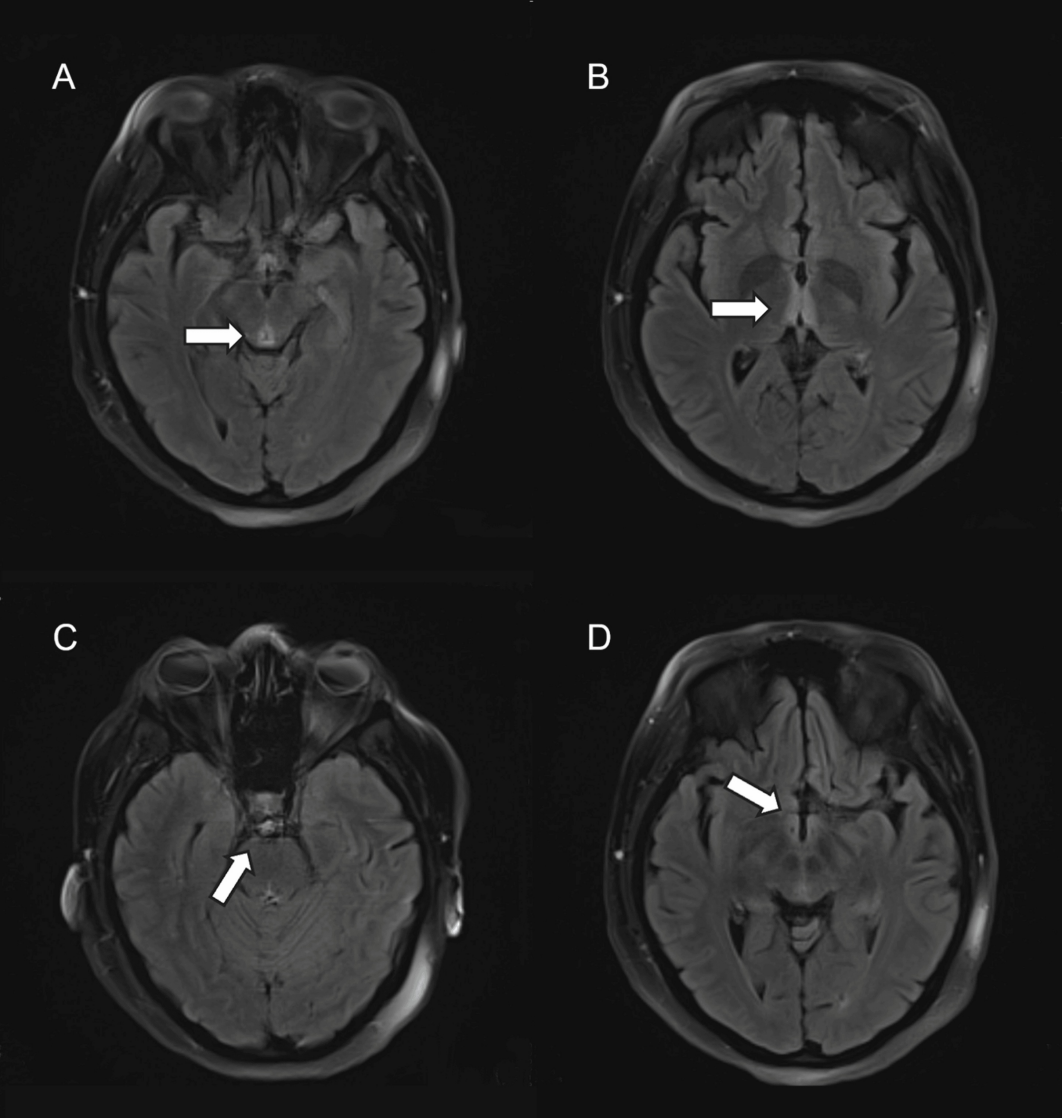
FLAIR MRI images showing hyperintense lesions (arrows) (A) Periaqueductal grey matter; (B) Dorsomedial thalamus; (C) Basis pontis; (D) Mammillary bodies FLAIR, fluid attenuation inversion recovery; MRI, magnetic resonance imaging
